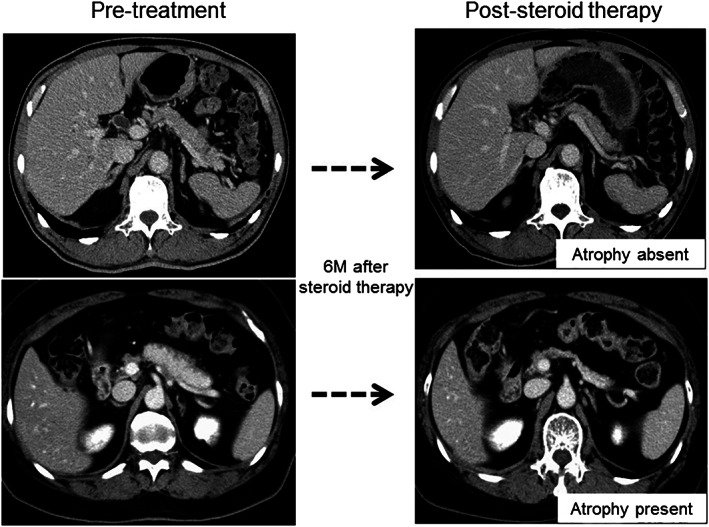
Typical computed tomography images of atrophy or nonatrophy cases before and after steroid therapy
